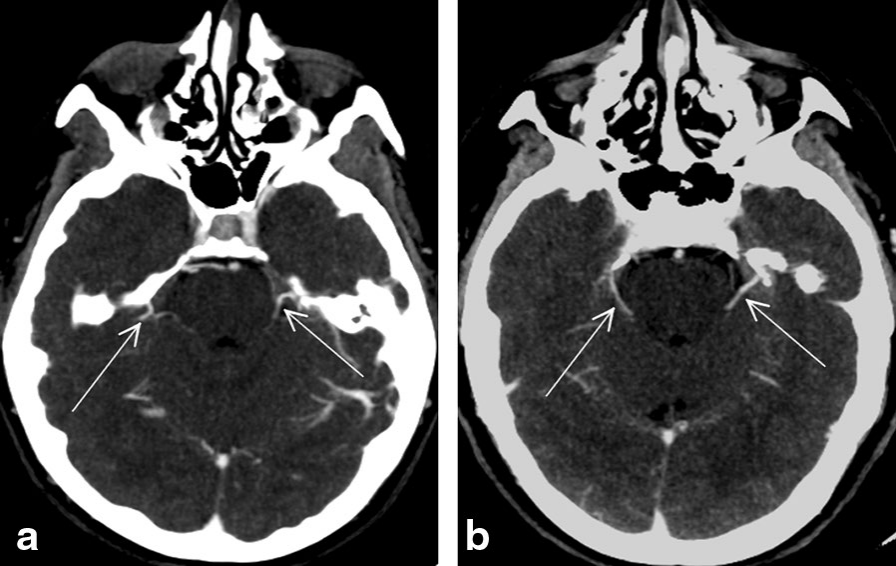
CTA axial views (a, b) of a control patient. *Arrows* indicate SPV
